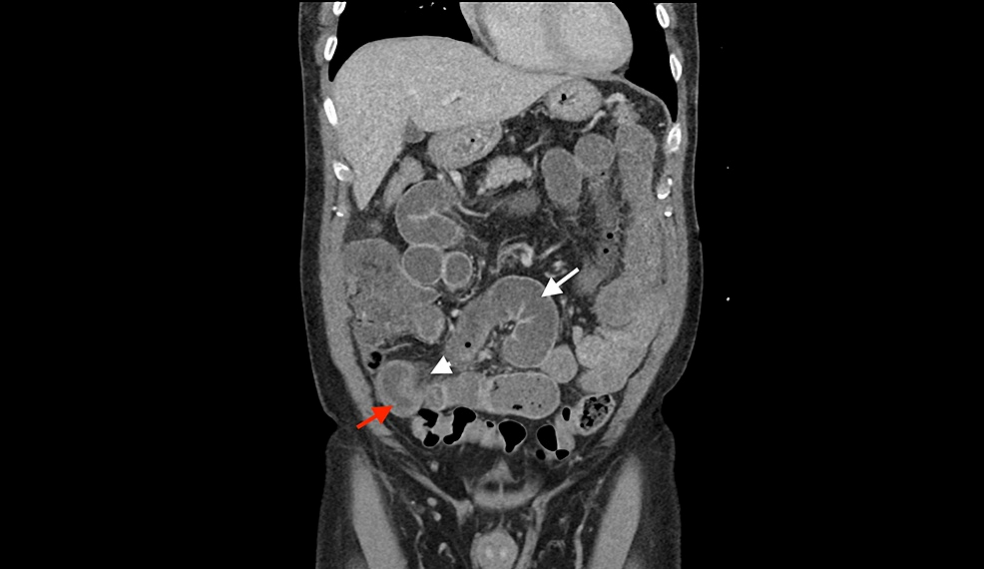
Computed tomography of the abdomen and pelvis: small bowel dilation measuring up to 3.5 cm in diameter (white arrow) with a transition point in the right lower quadrant where a wall thickening of the distal ileum (red arrow) is seen along with mesenteric fat stranding (white arrowhead). Findings are compatible with ileitis.
